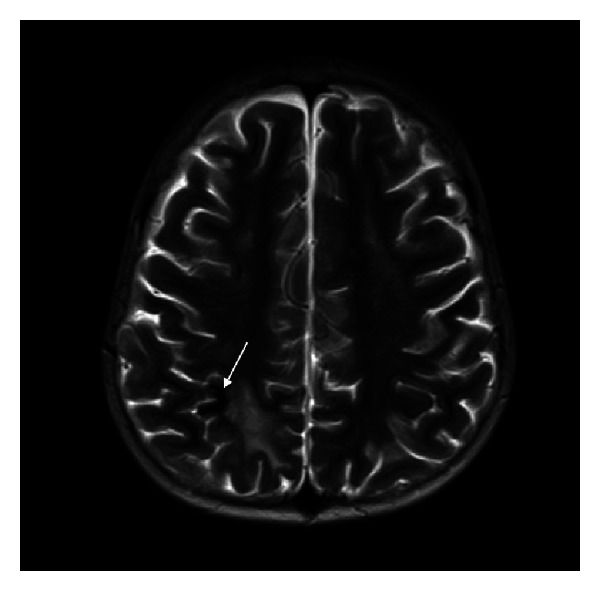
Axial T2-weighted MRI showing residual infarct with hemorrhage (arrow) in right frontal lobe with resolution of previous ischemic changes.
